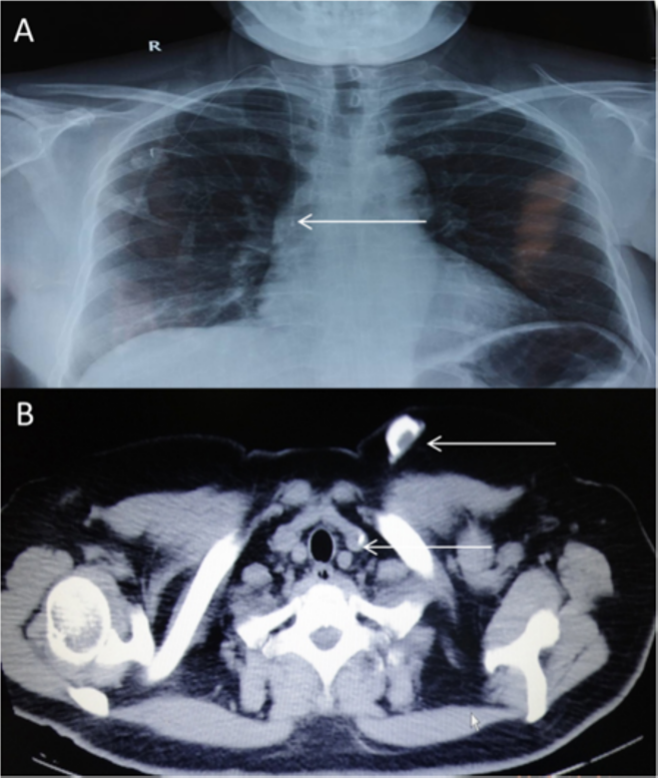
Catheter tip and port location. X-ray examination for catheter tip location (A). CT showing location of port and catheter (B).
